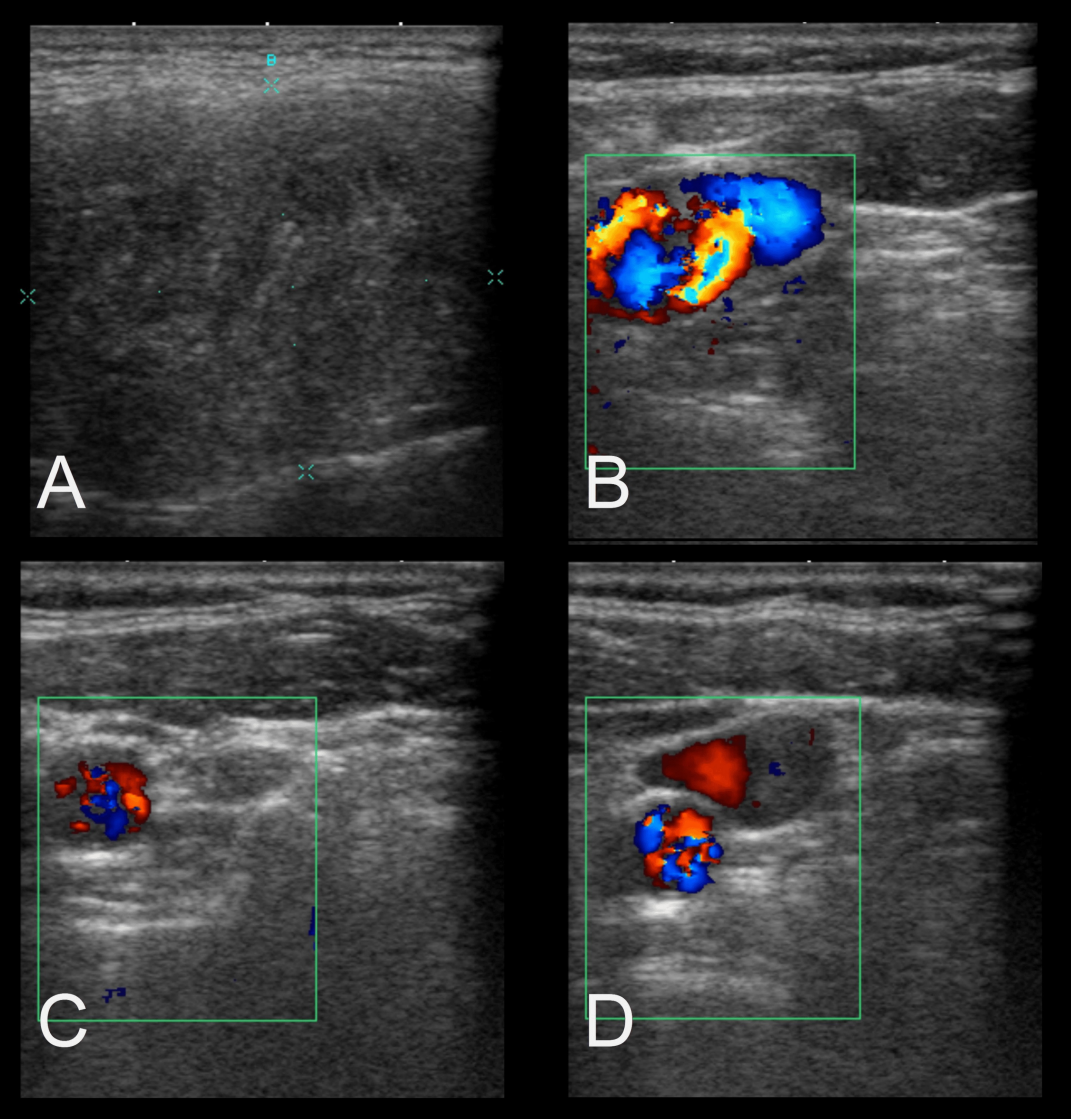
Neck ultrasound. (A) One hypo- to isoechoic nodular lesion at the left parotid gland, about 3.51 × 2.97 cm in size. (B, C, and D) The nodule demonstrates heterogeneous internal echotexture.
